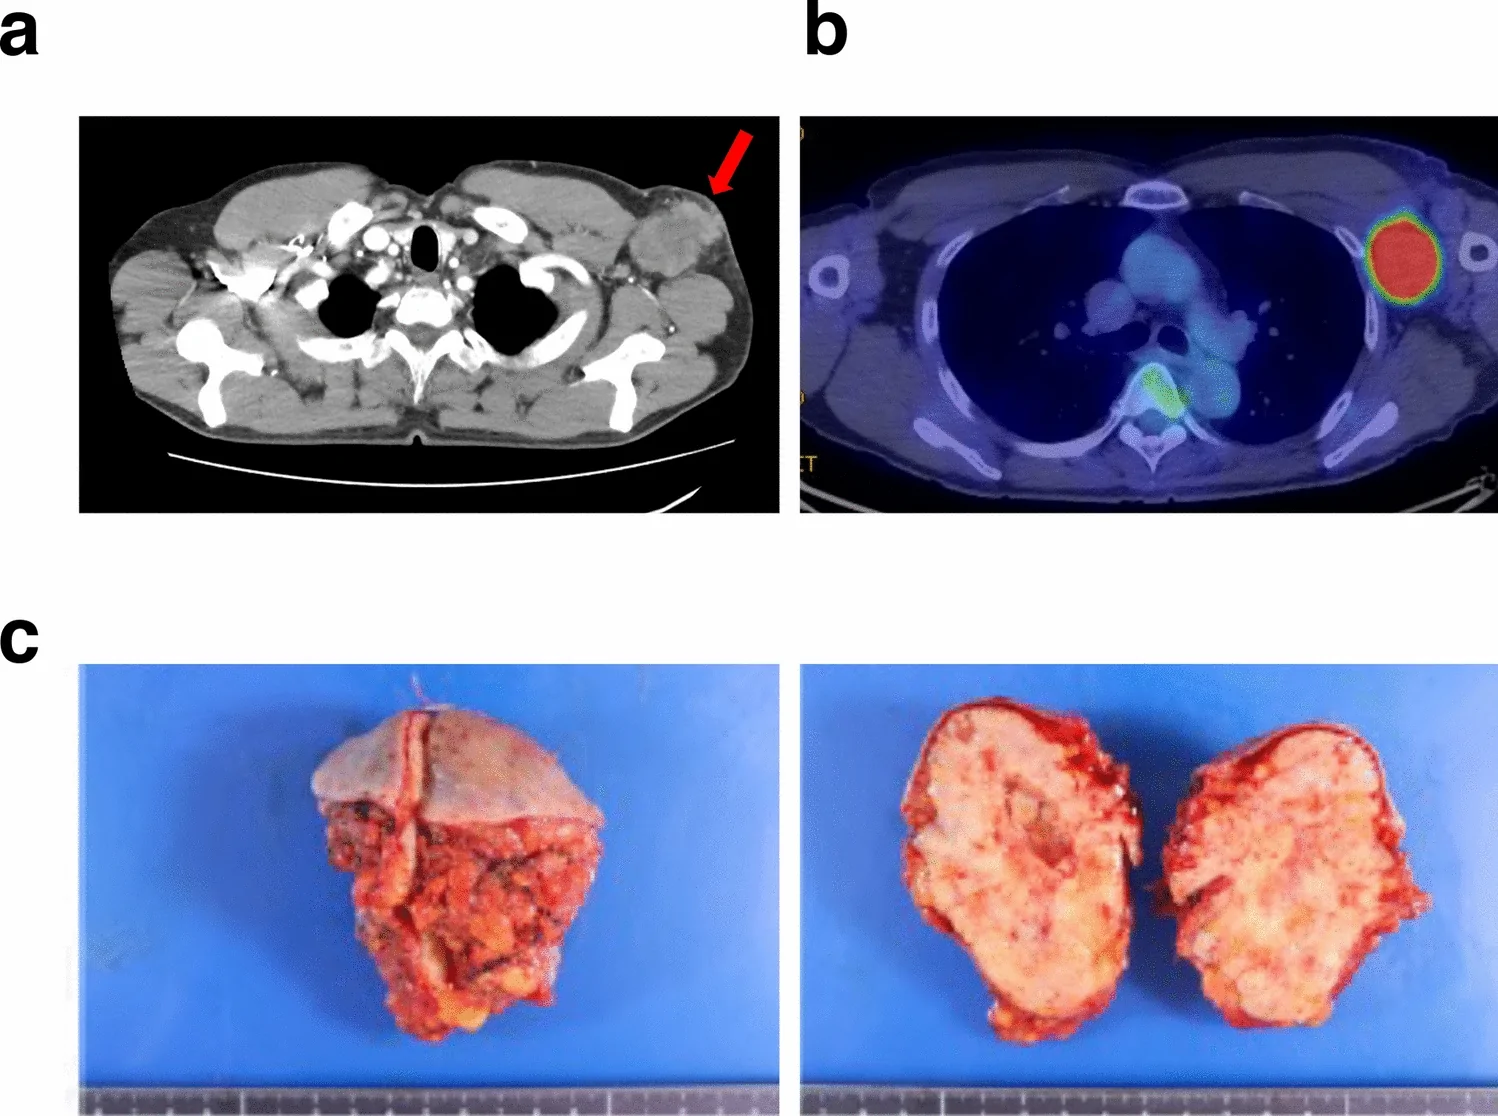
Clinical presentation of the patient with PDT-CUP#1. a CT revealed a mass with a maximum diameter of 4.5 cm in the left axillary region (red arrow). b PET-CT revealed intense FDG uptake within the mass. c Gross appearance of the resected tumor (left panel) and its cut surface (right panel)
